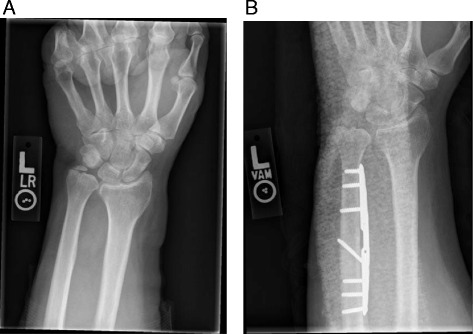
a PA radiograph of a patient with ulnar abutment revealing both 6 mm of ulnar-positive variance and incidentally an ulnar styloid nonunion. b Neutral to −1 mm of ulnar variance after a diaphyseal ulnar shortening osteotomy is performed
